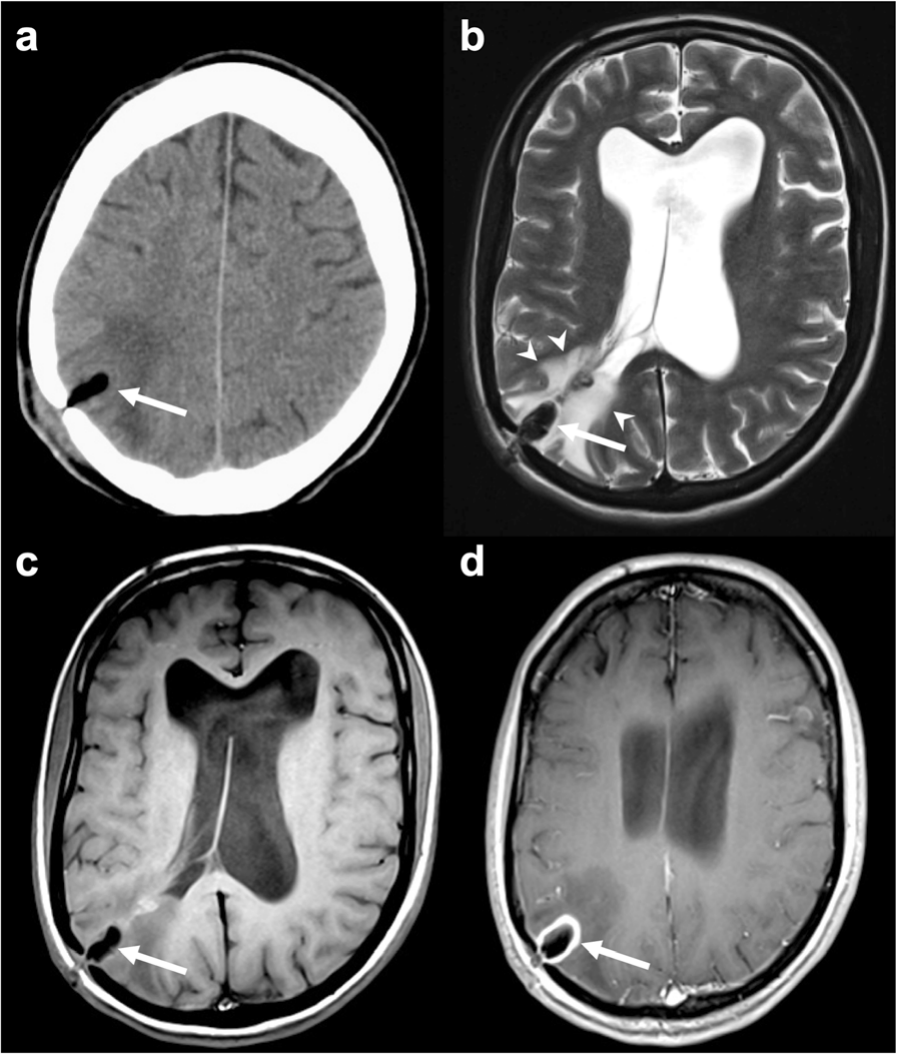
Bone wax granuloma. Axial unenhanced CT (a), T2-weighted (b), unenhanced (c), and post-contrast (d) T1-weighted images presenting an ovoidal mass (arrow in a, b, and c) hypointense on all sequences and surrounded by vasogenic edema (arrowheads in b). After contrast administration, rim enhancement is detectable (arrow in d)
